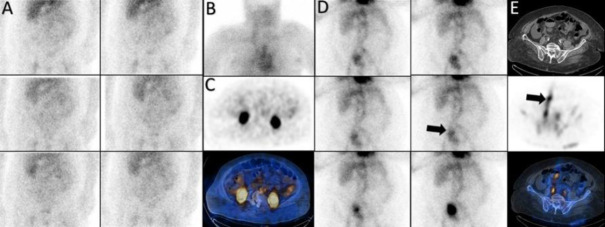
Dynamic images (A), cervico-thoracic spot image (B) and SPECT/CT images (C) in the first day showed sub-optimal with high background activity. In another day, dynamic images and SPECT/CT images showed the source of bleeding was sigmoid colon (black arrow, D-E)
